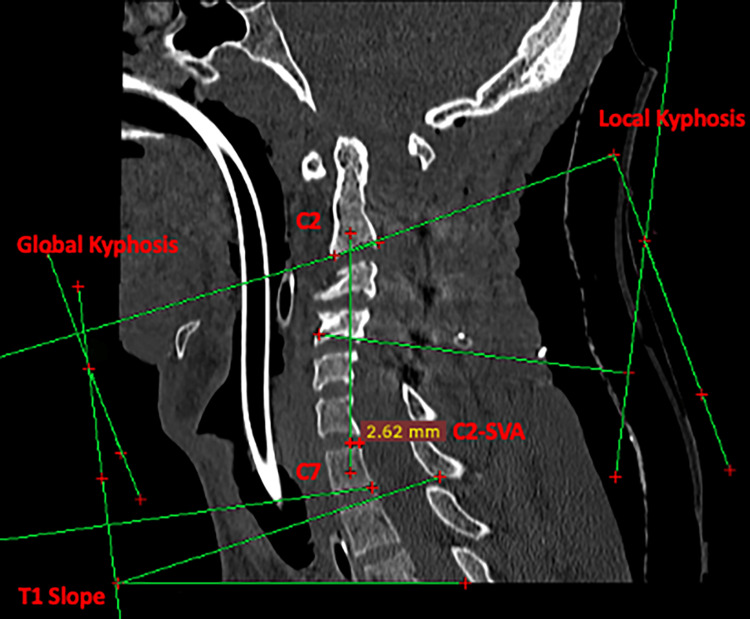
CT sagittal view showing post-operative assessment of various cervical parameters: local kyphosis 27.5 degrees, global kyphosis 14.4 degrees, neck tilt 43.6 degrees, T1 slope 18.2 degrees, and C2-SVA 2.62 mm. C2-SVA, C2 sagittal vertical axis
